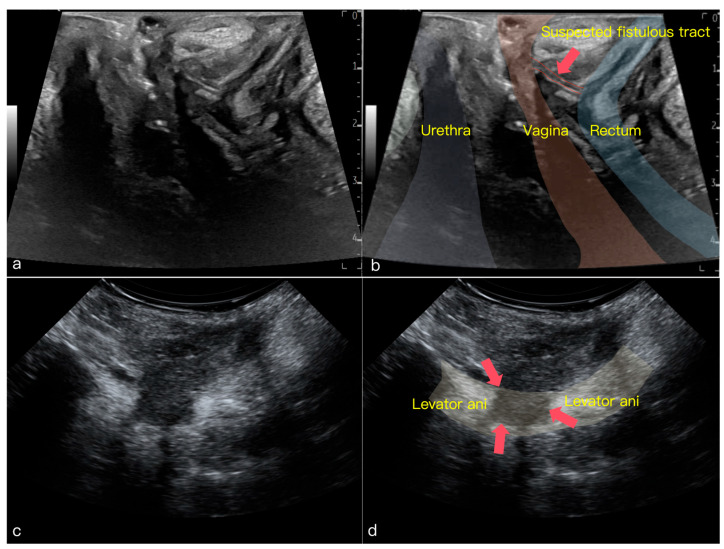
Typical findings of transperineal pelvic floor ultrasound in rectovaginal fistula (RVF). (a) Mid-sagittal TPUS image showing the anatomical relationship between urethra, vagina, and rectum (unannotated). (b) The same mid-sagittal image with annotations; the red arrow indicates a suspicious fistula tract between the vagina and rectum. (c) Parasagittal TPUS image depicting the morphology and continuity of the levator ani muscle (unannotated). (d) The same parasagittal image with annotations; the red arrow indicates a discontinuity in the hypoechoic muscle ring, consistent with levator ani injury.
